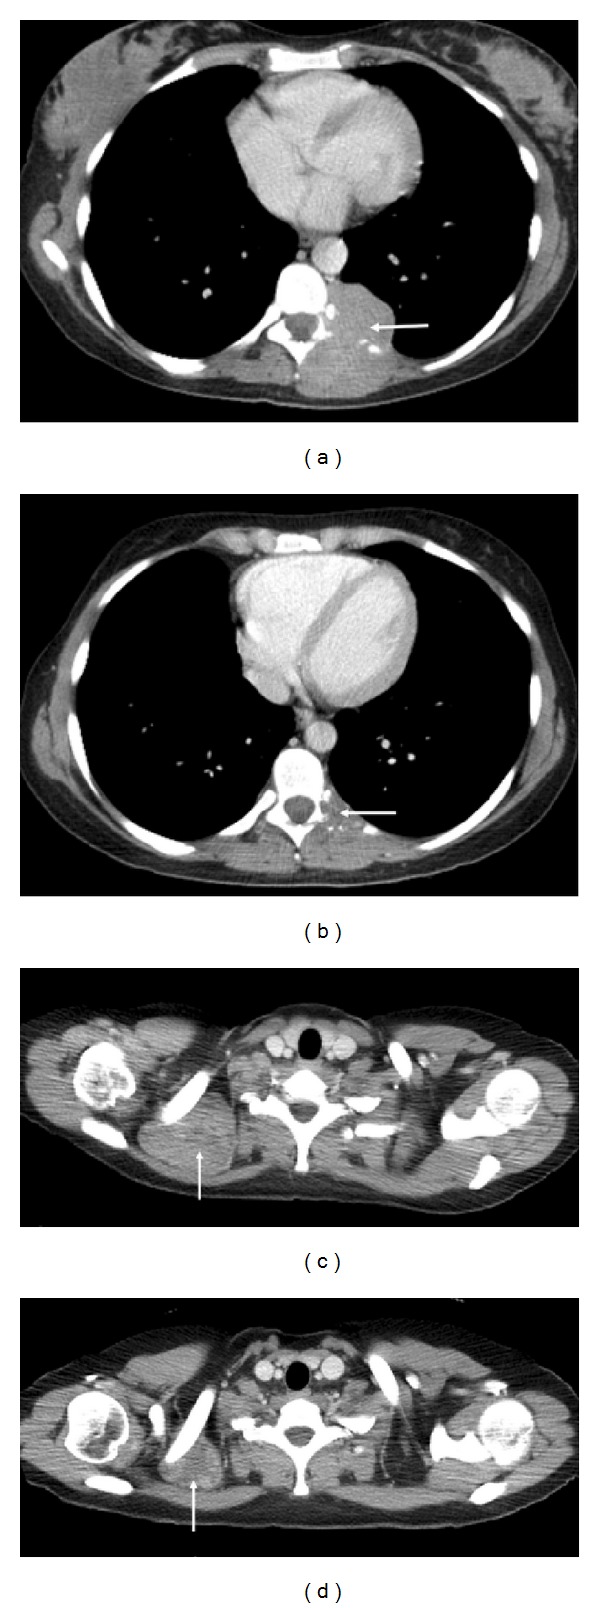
Axial contrast enhanced CT images of the lower and upper thorax in a 37-year-old female with metastatic synovial sarcoma at baseline ((a) and (c)) and following 2-month therapy ((b) and (d)). The 5.6 cm left sided paravertebral mass which was causing destruction of the posterior rib ((a), arrow) showed a dramatic reduction in size with only a thin plaque of indeterminate low attenuation tissue remaining at this site ((b), arrow). A further site of disease behind the right clavicle ((b), arrow) reduced in size from 6.5 cm to 3.6 cm ((d), arrow).
